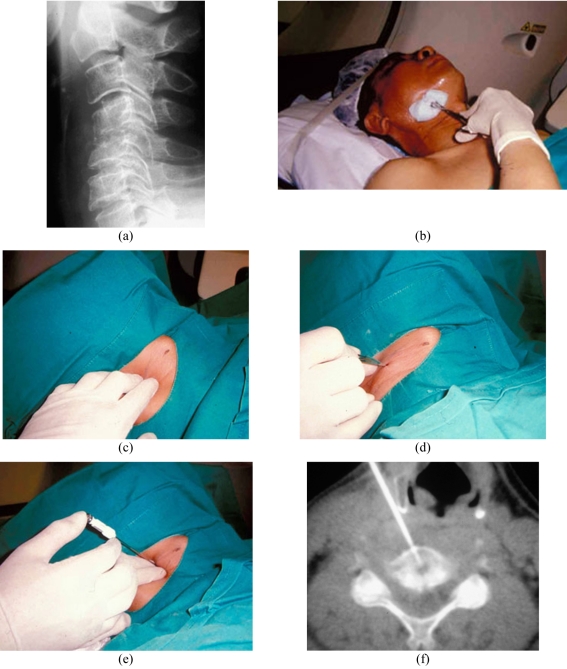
Biopsy of the C4/5 infective spondylodiscitis in a 50-year-old man using the anterolateral approach. (a) Lateral radiograph shows irregular narrowing of the C4/5 disc space with mild anterior wedging of C4 and C5 vertebral bodies and mild kyphotic deformity. (b) Photograph shows the patient lying in a supine position with the neck being cleaned after the selected level was surface-marked. (c) Photograph taken after the patient was draped shows the radiologist’s fingers palpating the carotid pulsation and guarding the right major neurovascular bundle. (d) Photograph shows the skin incision being made following administration of local anaesthetic. (e) Photograph shows insertion of the Ostycut biopsy needle. Note that the radiologist’s fingers are guarding the adjacent major neurovascular bundle during needle insertion. (f) CT fluoroscopic image taken with the patient lying supine shows the tip of the Ostycut needle within the inferior C4 vertebral body osteolytic lesion.
